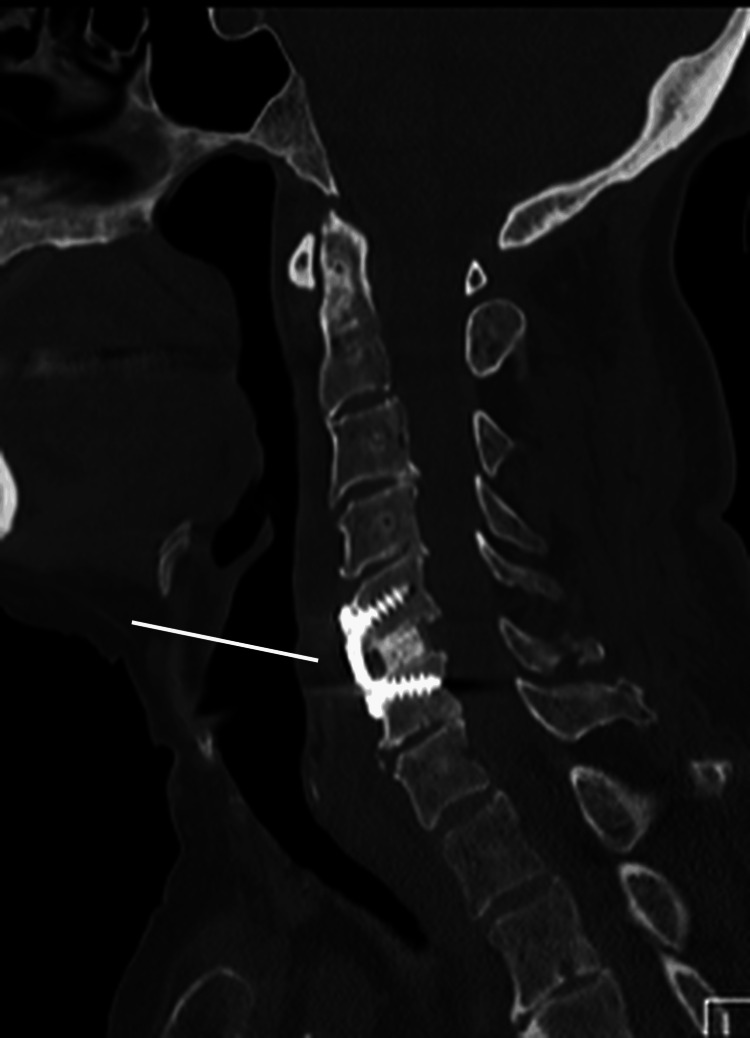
Sagittal CT scan of the cervical spine showing anterior fixation at C5 and C6 levels and position of metalwork and vertebral alignment. CT: computed tomography, C5/C6: cervical vertebrae 5/6.
